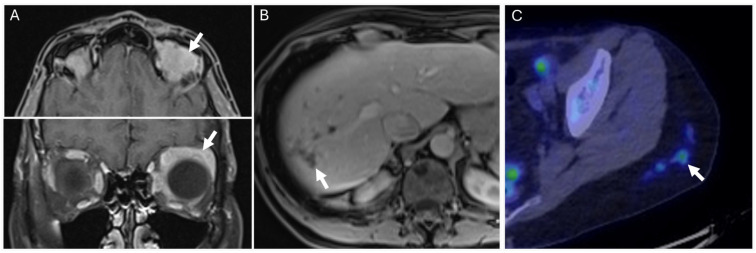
A 59-year-old female with rapid growth of an eyelid lesion. MRI of the orbit (A, arrows) with a plaque-like mass in the superomedial left preseptal orbit. Biopsy was consistent with NXG. Further workup revealed transaminitis and MRI abdomen (B, arrow) revealed linear and nodular areas of hypoenhancement in the right lobe of the liver. Biopsy was positive for NXG. PET CT revealed additional nodules, such as in the subcutaneous soft tissues of the left posterolateral thigh (C, arrow) with SUV max 6.1, which were also biopsied and positive for NXG. The patient was also found to have IgM monoclonal gammopathy.
